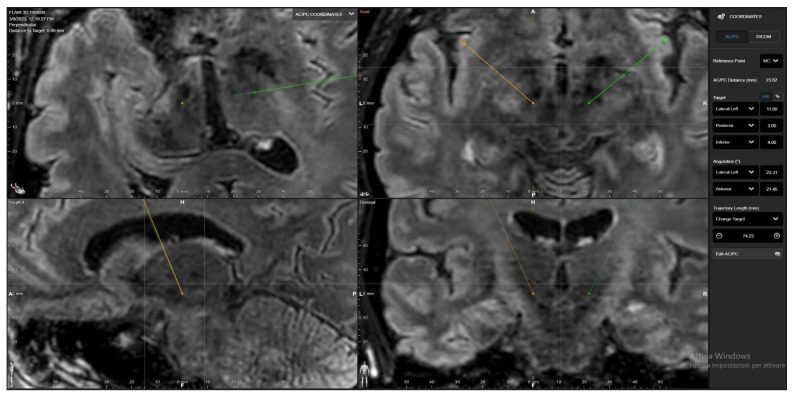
Conventional targeting using Schaltenbrand and Wahren [6] coordinates adjusted with 3T MRI imaging.
